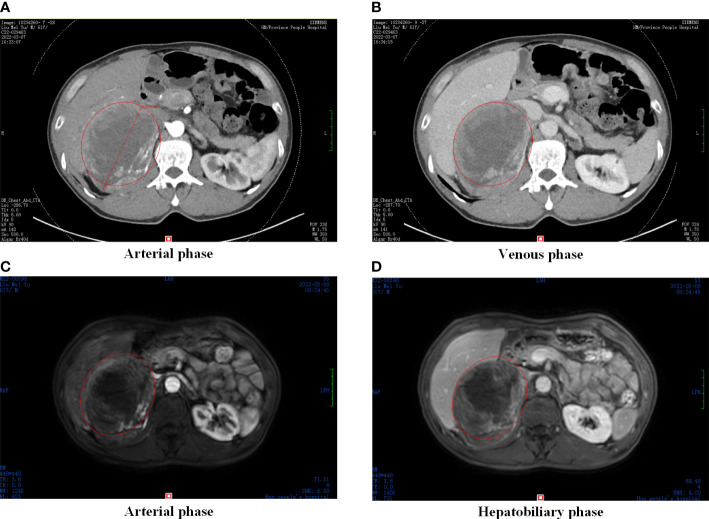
Preoperative abdominal CT and MRI. (A) CT scan in arterial phase, (B) CT scan in venous phase; (C) MRI scan in arterial phase, (D) MRI scan in hepatobiliary phase. Abdominal CT and MRI images showed an oval and smoothly mass located in the liver-kidney space (red circle).
